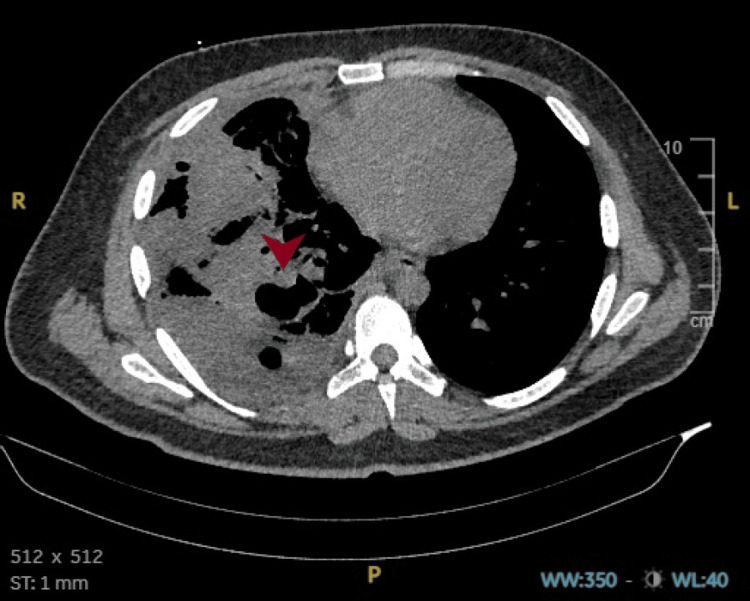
CT aortogram in mediastinal window demonstrating a Rasmussen aneurysm (arrowhead). CT: computed tomography
